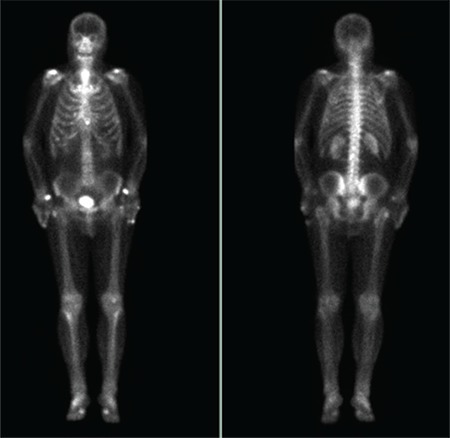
Bone phase images were consistent with stable degenerative changes and bone metastasis was not observed. Physical examination of the patient revealed bilateral painful gynecomastia. In the drug questionnaire, it was learned that the patient was given Bicalutamide therapy after the first bone scan. No other drug use or any disease that might cause gynecomastia was found. Bicalutamide is a nonsteroidal drug used in patients with prostate cancer, which can be administered in combination with LHRH analogues. It has a competitive inhibitor effect on cytosolic androgen receptors in the target tissue (1,2). Gynecomastia, breast pain, fatigue and decreased libido are common side effects of Bicalutamide (3,4). When an increase in estrogen/androgen ratio in the breast tissue occurs, this may cause gynecomastia and breast pain (5). When inflammation or swelling occurs in breast regions, this may be seen on blood pool images. If these hyperemic tissues or lesions have no effect on nearby bones, clearance of the radiopharmaceutical happens and normal distribution of the tracer is seen on late bone phase images. We routinely perform 2-phase imaging in oncology patients to analyze the possible hyperemic status of bone lesions for the differential diagnosis of benign or malignant pathologies. Physical examination may help narrowing the differential diagnosis among gynecomastia, other soft tissue lesions or radioactivity contamination, when this sign is seen. It is also important to keep in mind that gynecomastia can sometimes be seen unilaterally. There are also several reports in the literature about incidental gynecomastia and other nuclear medicine procedures apart from bone scan, such as sodium fluoride positron emission tomography (PET), myocardial single photon emission computed tomography or single photon emission computerized tomography PET imaging (6,7,8). Blood pool images may detect this side effect and should be evaluated with physical examination in case of clinical doubt.
